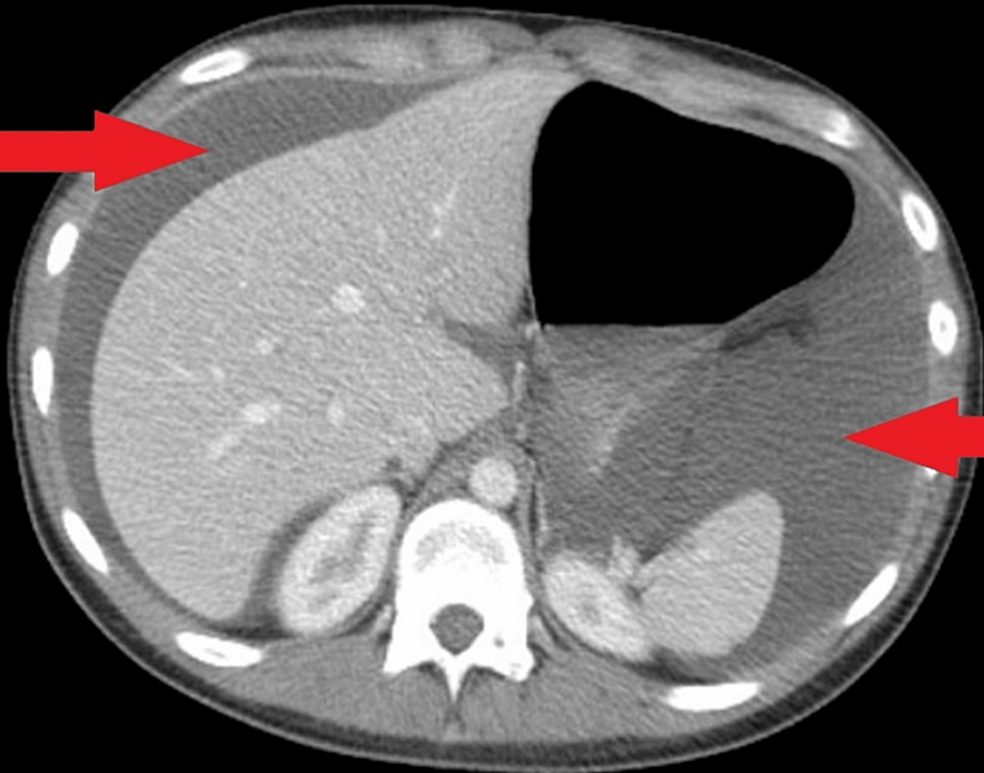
Axial CT of the abdomen/pelvis shows ascites (red arrows).
